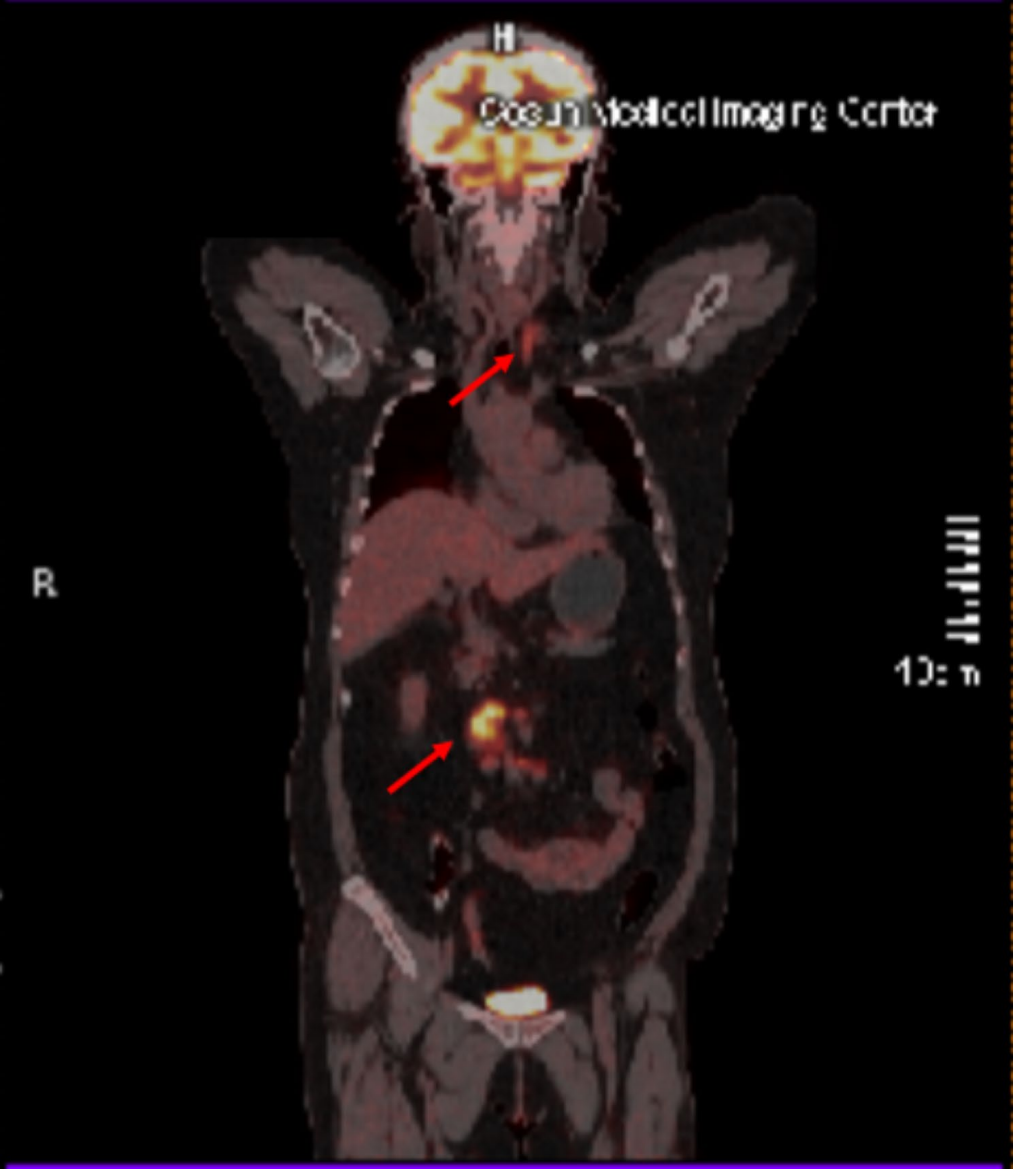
PET-CT scan showing left cervical and supraclavicular lymphadenopathy with retroperitoneal lymphadenopathy (arrows)
